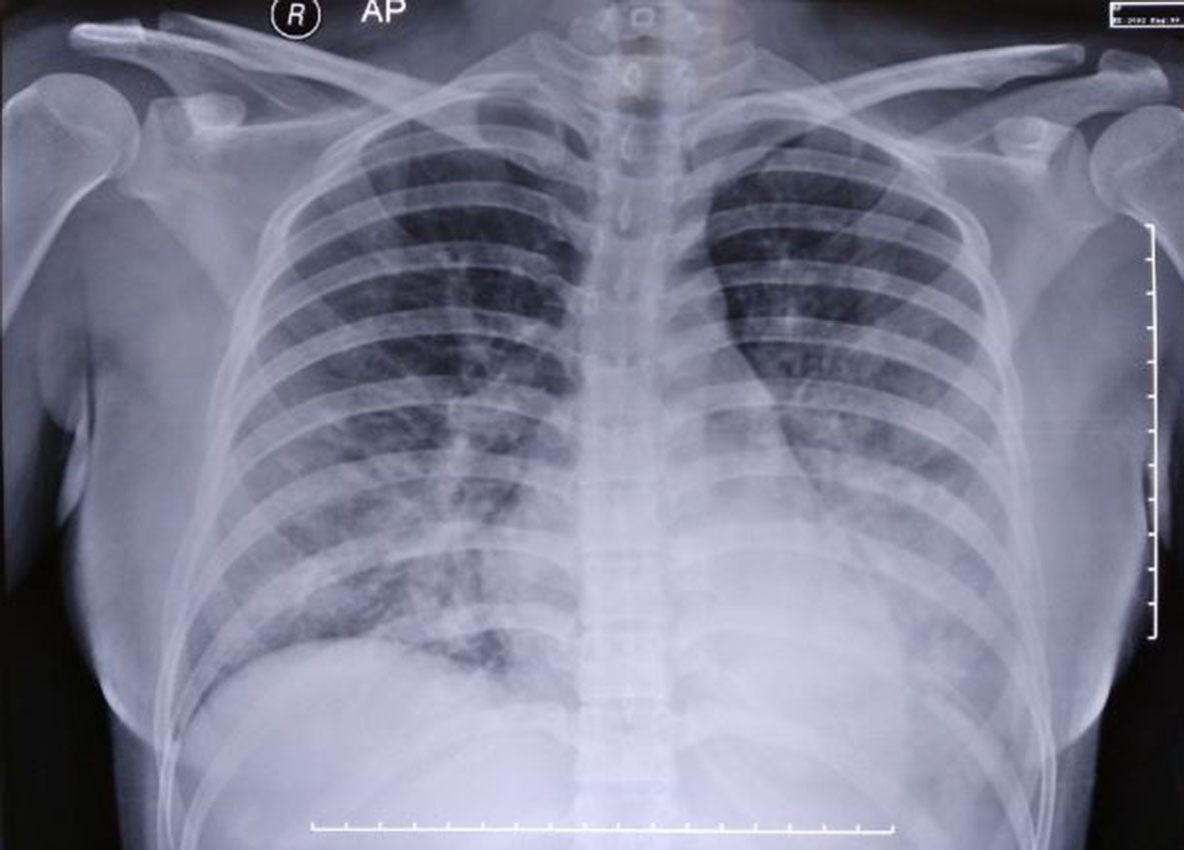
Chest X-ray upon initial admission
